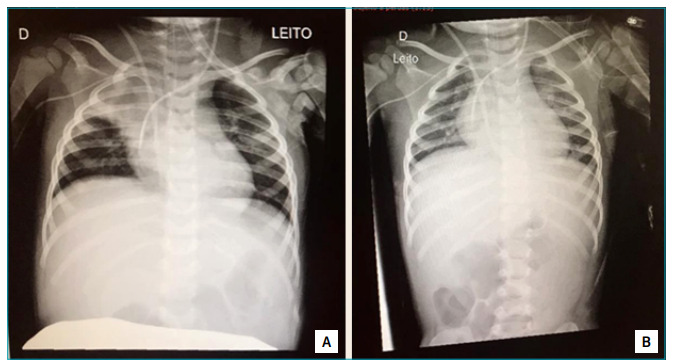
Chest radiography of patient with epidermolysis bullosa. (A) Hypotransparency at the right apex; (B) Improvement of hypotransparency after expiratory pause maneuvers during closed system aspiration.
